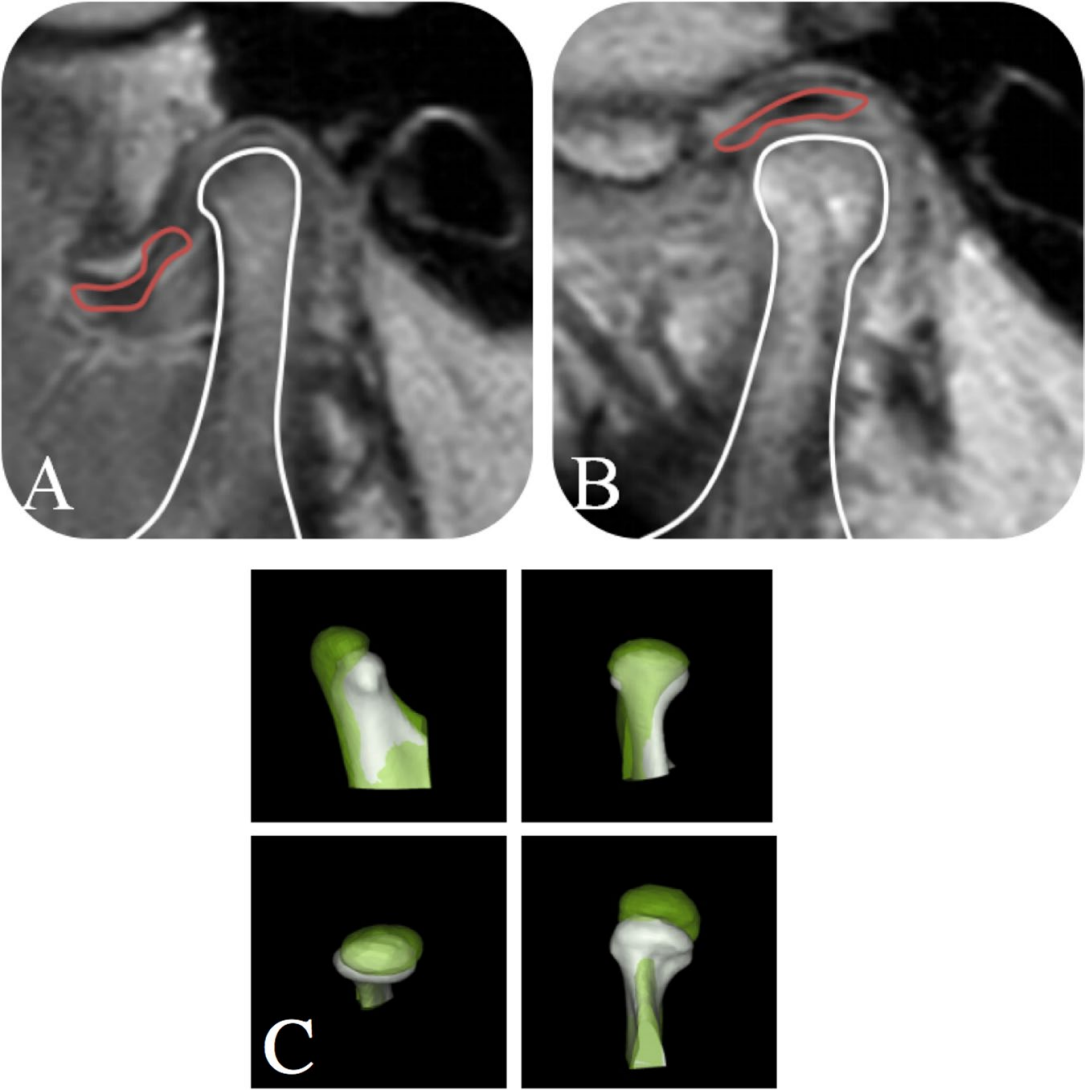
MRI of ADDWoR before treatment (A); MRI of ADDWoR 12 months after arthroscopy surgery (B); 3D volume-rendering images showed achievement of vertical and horizontal bone augmentation (C).
